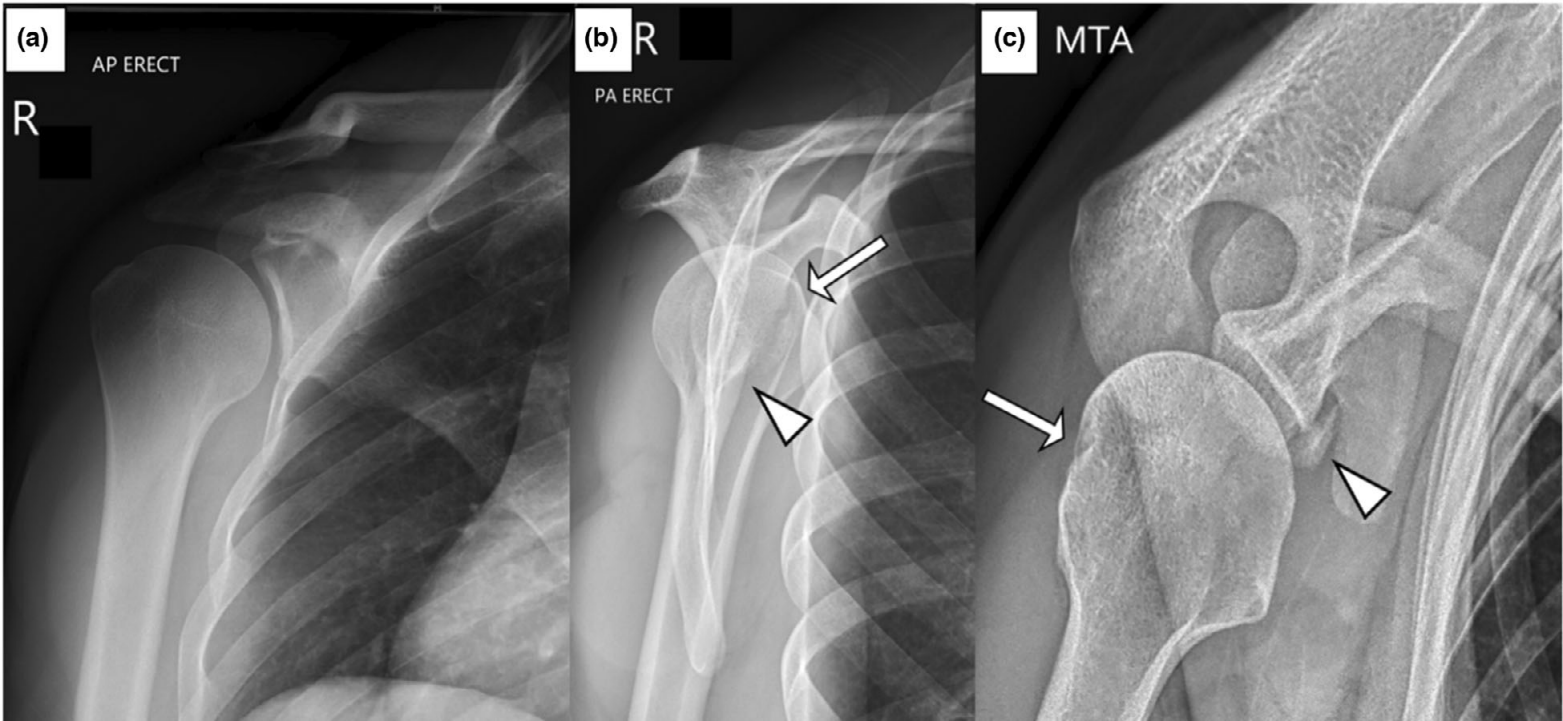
The patient presented to emergency department after a shoulder reduction attempt in the ambulance and no radiographs were taken prior. Glenoid view (A) has no abnormality detected. The lateral scapula shoulder projection (B) has a subtle radiolucency line in the anterior glenoid rim (arrowhead), suggesting a bony Bankart. The MTA view (C) confirms the bony Bankart (arrowhead) and the depression of the posterolateral aspect of the humeral head is the Hill‐Sachs lesion (arrow). MTA, Modified trauma axial.
